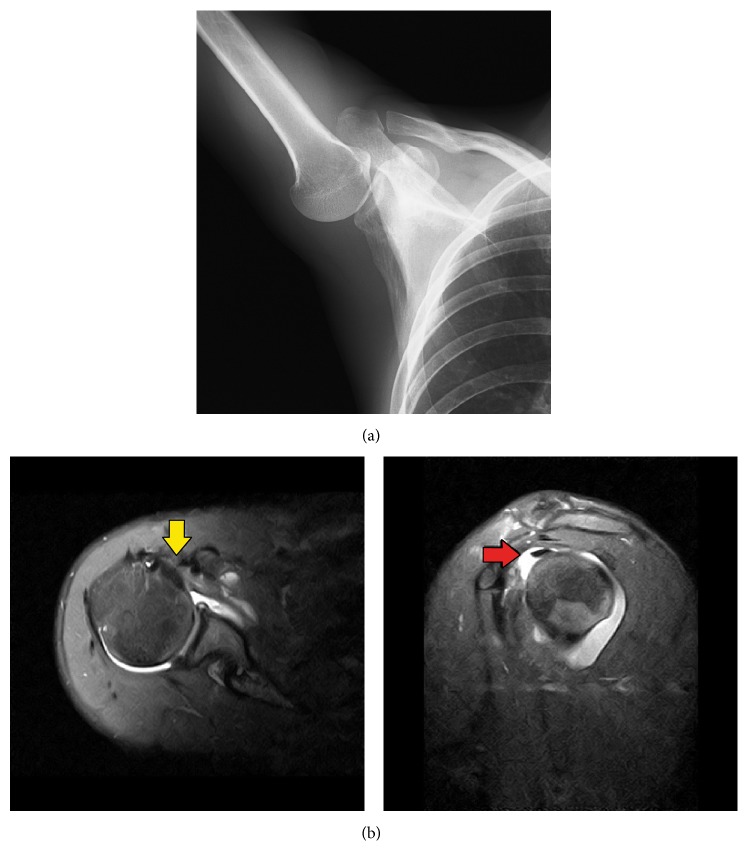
(a) X-ray image of the upper arm in the elevated position showing humeral head slipping. (b) MRI images of the superior aspect of the subscapularis tendon and muscle injury (yellow arrow) and rotator interval lesion (red arrow) (fat suppressed imaging).
